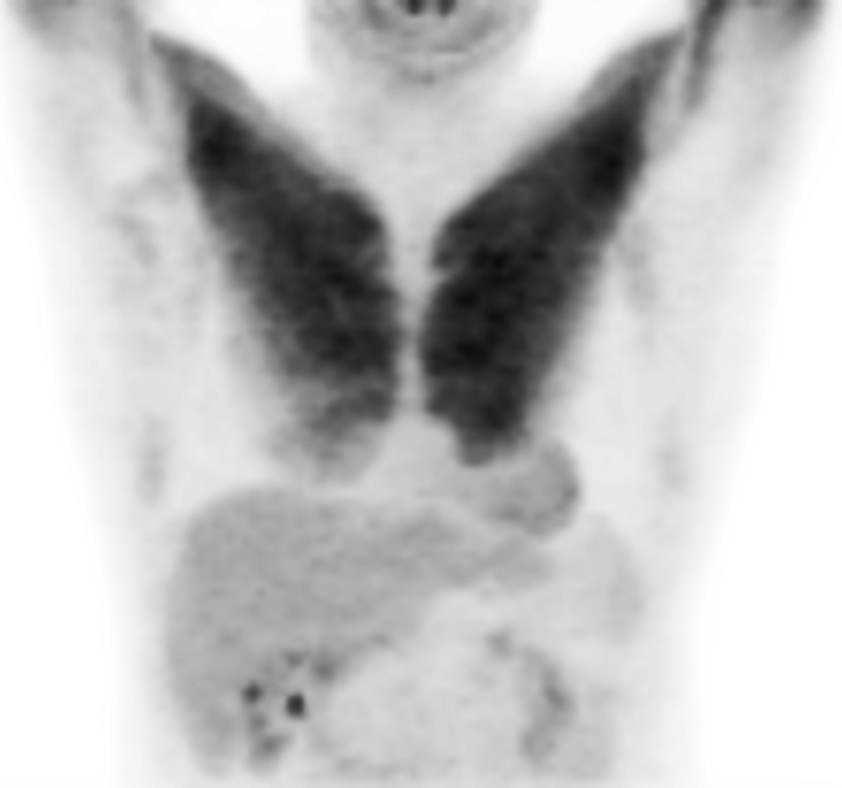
FDG PET scan (coronal view. Grade 3 to 4 symmetrical F-18 FDG uptake of the pectoralis major muscle) [Image courtesy of Centre for Molecular Imaging, Peter MacCallum Cancer Centre, East Melbourne, Victoria, Australia].
